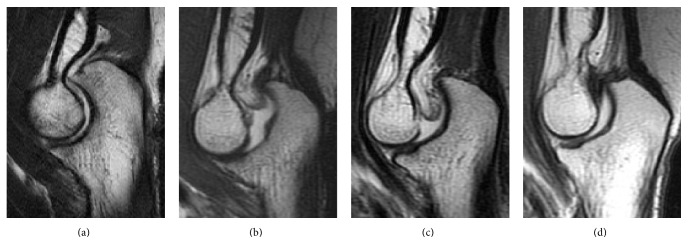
Magnetic resonance images of the elbow. (a) Sagittal T2-weighted image of a normal elbow. Preoperative sagittal T2-weighted images of the (b) right and (c) left side in the present case show a hypertrophied posterior fat pad interposed between the olecranon and olecranon fossa in both elbows. The posterior fat pad in the left elbow is located more inferiorly than that in the right elbow. Postoperative sagittal T2-weighted image of the (d) left elbow shows no posterior fat pad interposed between the olecranon and olecranon fossa.
